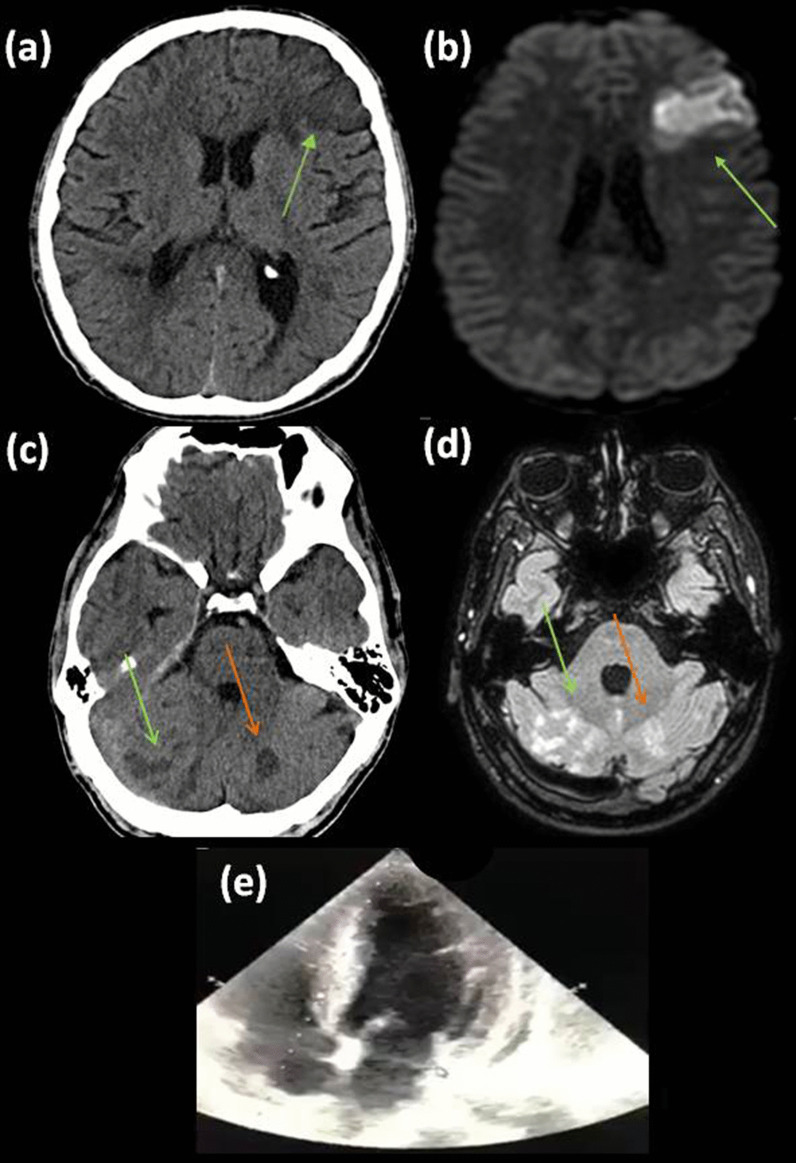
Post-IVT CT and DWI images demonstrating appearance of a new hypodense lesion (a) exhibiting true restriction (b) respectively, extending over an area irrigated by the superior trunk of the left MCA. Multiple new hypodense (CT)/high signal intensity lesions (FLAIR) within the right cerebellar hemisphere (PICA territory) and vermis (green arrows) and an older infarcted area on the left (orange arrows) (c, d). TTE exhibiting full LVT lysis (e)
